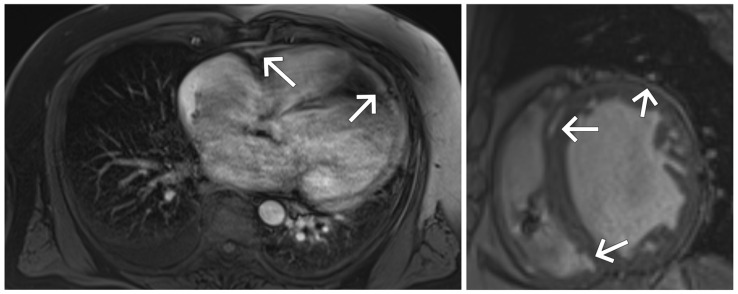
Cardiac magnetic resonance imaging: markedly increased ventricular volumes, and diffuse non-ischemic late gadolinium enhancement of both ventricles shown in long-axis (left panel) and short-axis (right panel) views. LGE involves subendocardial, mid-wall, and subepicardial layers, consistent with diffuse granulomatous infiltration. White arrows indicate the areas of late gadolinium enhancement.
